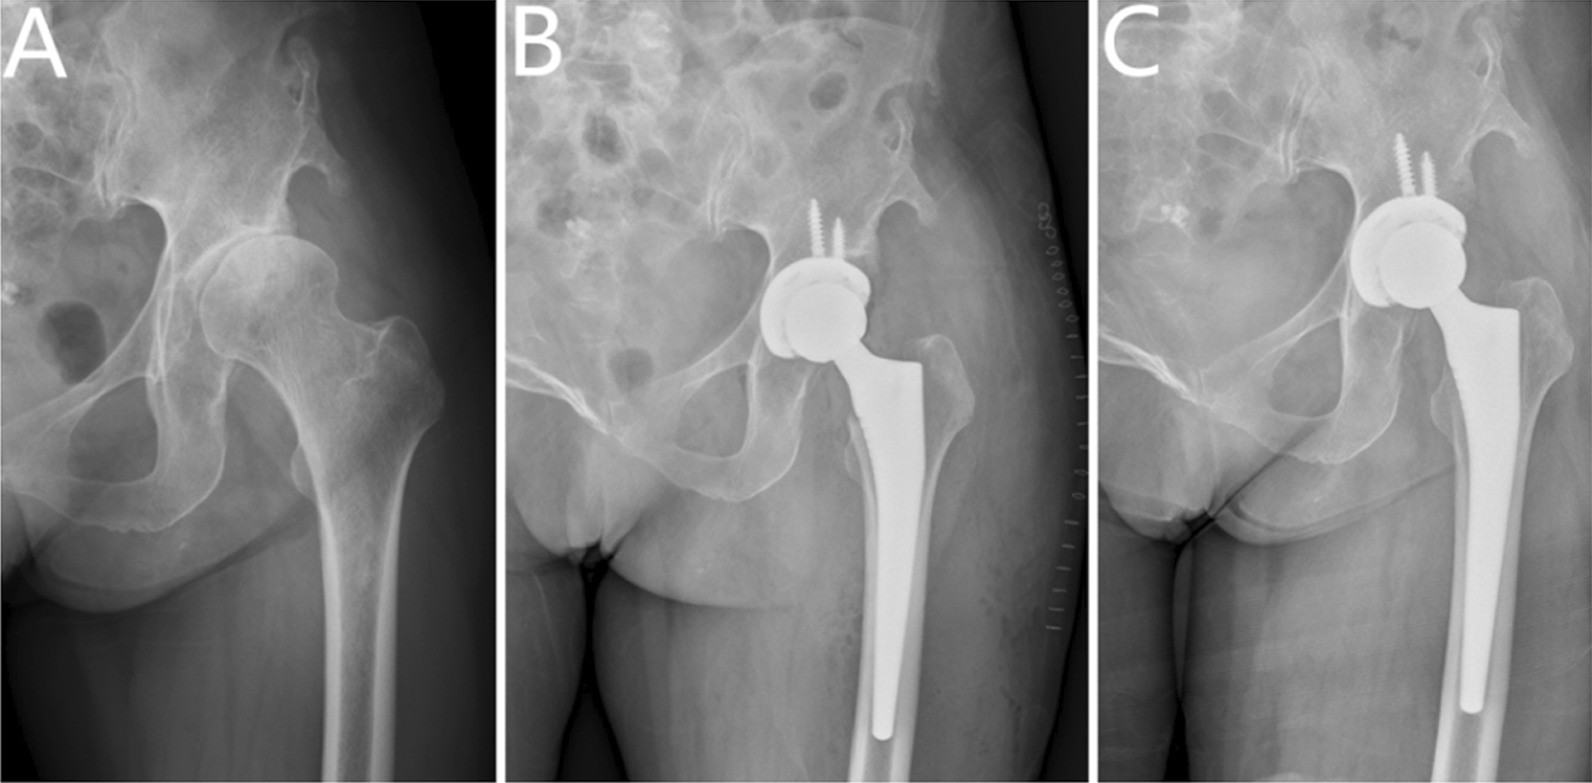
A Preoperative radiograph of the hip of a 56-year-old female with residual poliomyelitis,showing a osteoarthritis secondary to developmental dysplasia of the hip. B postoperative imaging. C Radiograph obtained 4.7 years after surgery,showing no evidence of implant subsidence,implant loosening or osteolysis
